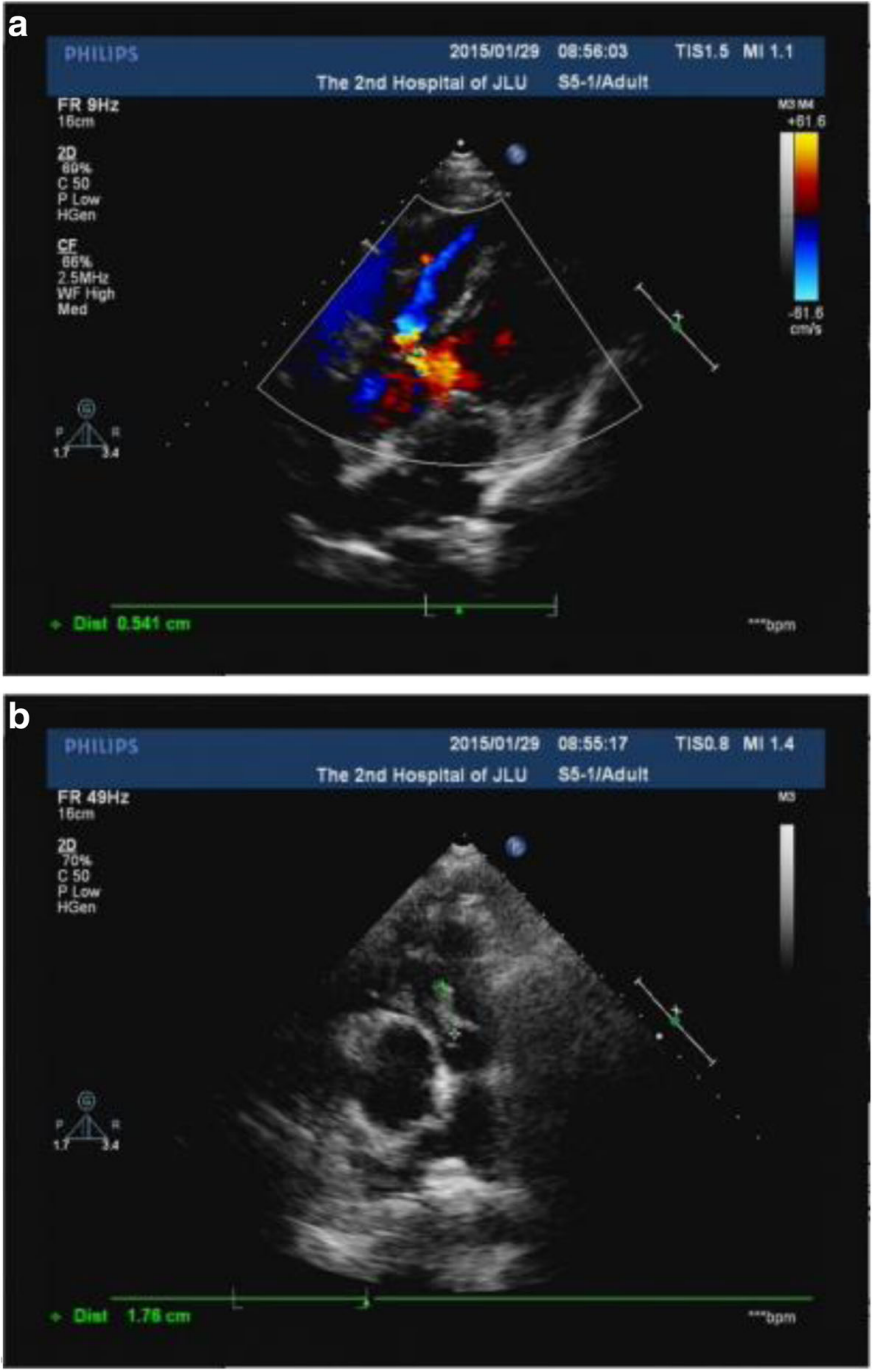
a Transthoracic parasternal short axis view showing vegetation (white arrow) on pulmonary valve. b Transthoracic echocardiography showing a small perimembranous type VSD with left to right shunt
